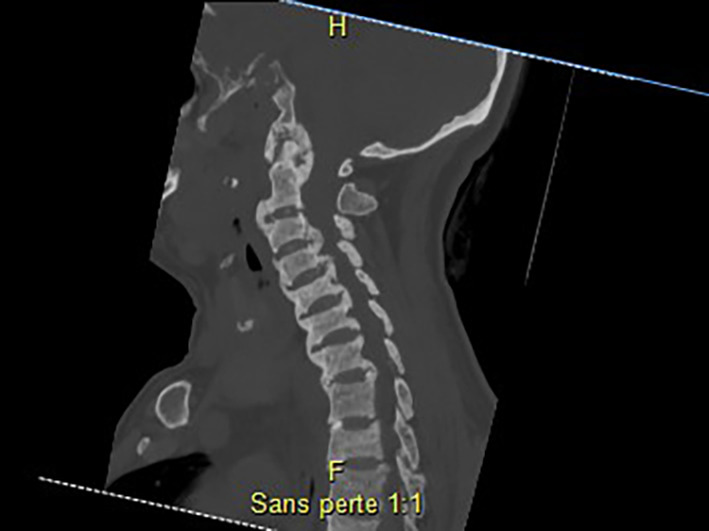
CT scan of the cervical spine (March 2017). Sagittal sections: diffuse bone sclerosis, predominant on the vertebral bodies at the cervical level, sites of biconcave compression. Hyperostosis with enthesopathy and more pronounced discal‐ligamentous ossifications on the anterior and posterior longitudinal ligaments. Ossification of the annular ligament with a “crowned dens” appearance. C_0_–C_1_ and C_1_–C_2_ degenerative modifications.
